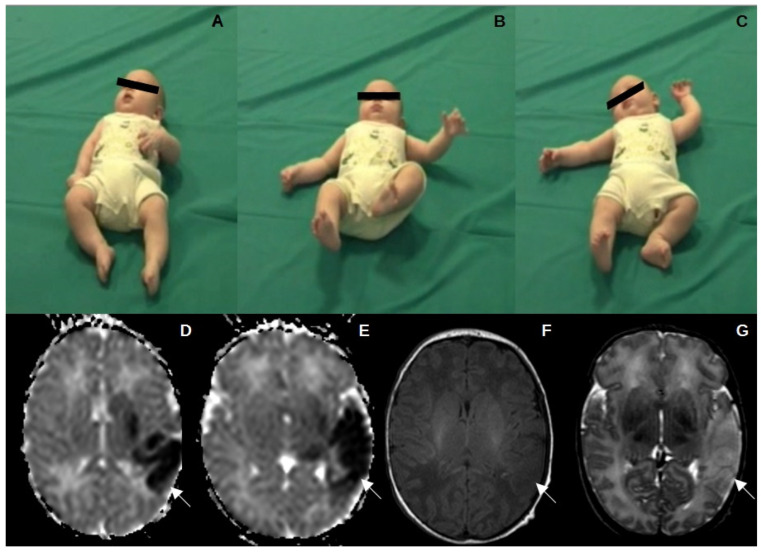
Absent FMs, SMs, and postural asymmetry (A–C) in a patient (case 15) with left MCA stroke, who developed right UCP. Cerebral MRI performed at 5 days of life showed diffusion-weighted (D,E), T1 (F), and T2 (G) abnormalities in the left MCA cortical and subcortical areas.
